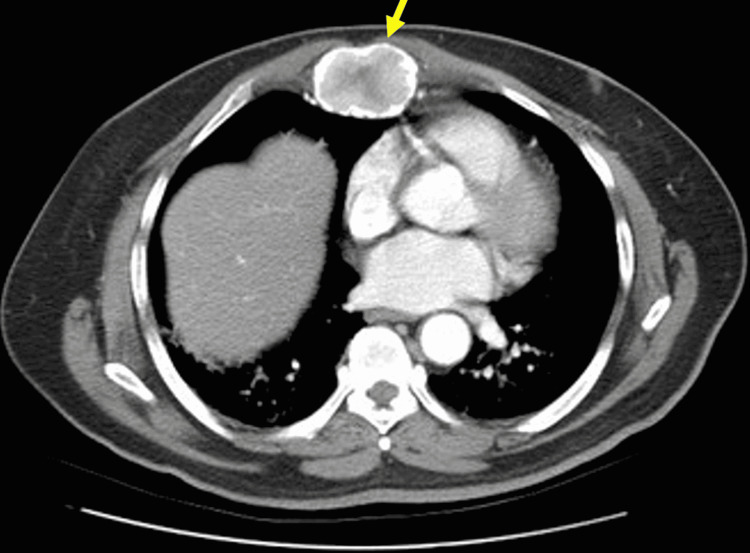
Axial computed tomography (CT) image of an osteolytic sternal mass
